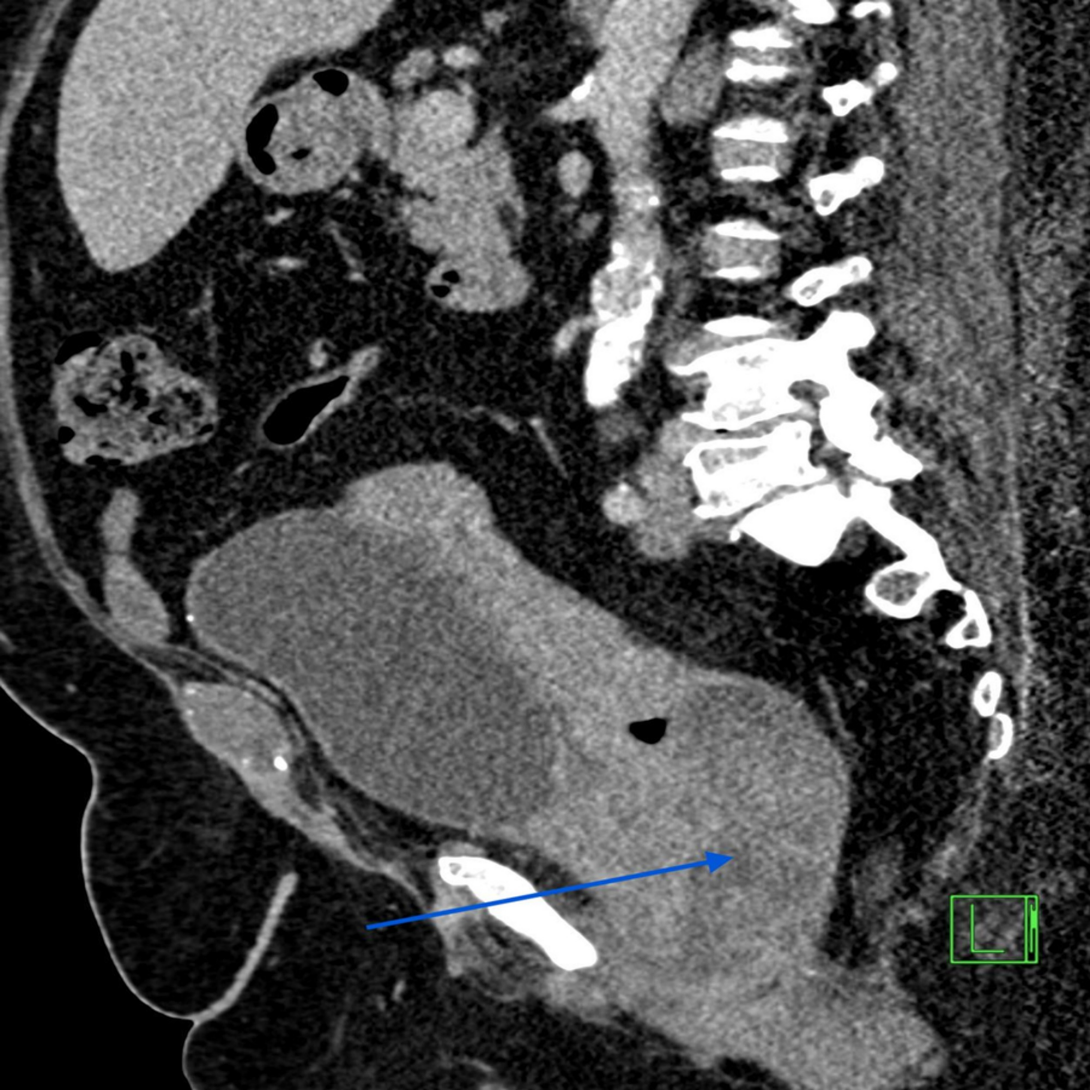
Sagittal section of the CT scan of the abdomen and pelvis showing the tumor mass in the upper vagina (arrow)
